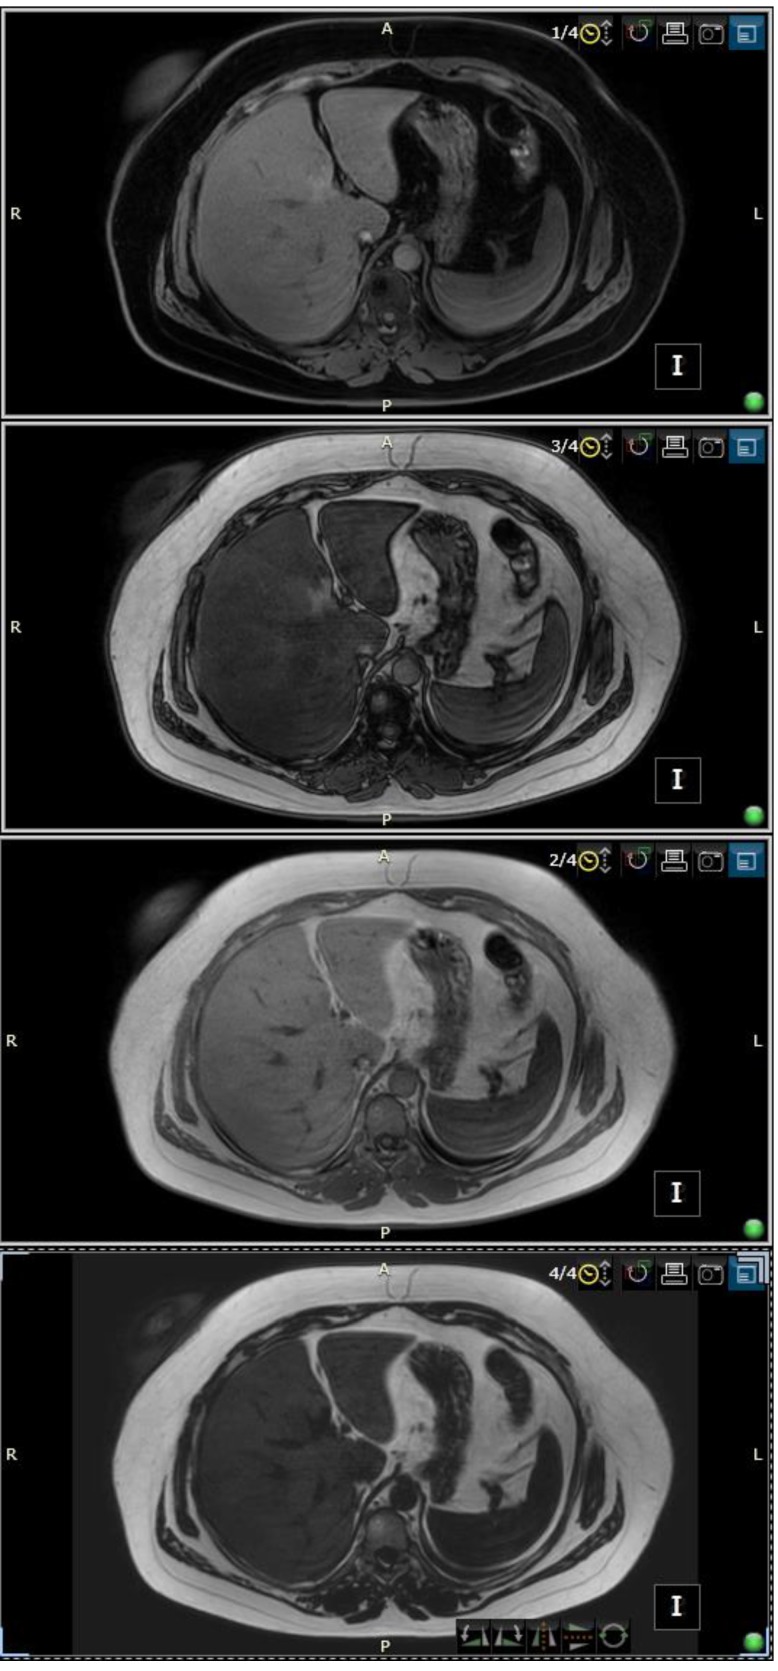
3T MRI, multi-echo DIXON-All breath hold sequences consisting in T1-weighted sequence, in-phase T1 weighted sequence, out-of-phase T1 weighted sequence and fat specific sequence qualitatively assessing liver steatosis
